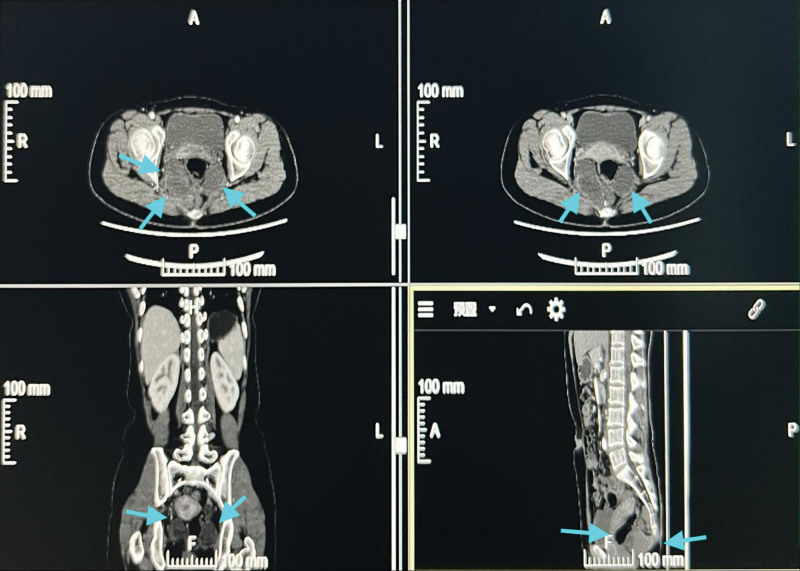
Enhanced CT showed multiple multilocular cystic increased density shadows in the sacrococcygeal region, which were irregular in shape and clear in border, calcified shadows were observed at the edge of some lesions, and the surrounding fat space was clear, which was considered to be cystic mature teratoma. CT = computed tomography.
